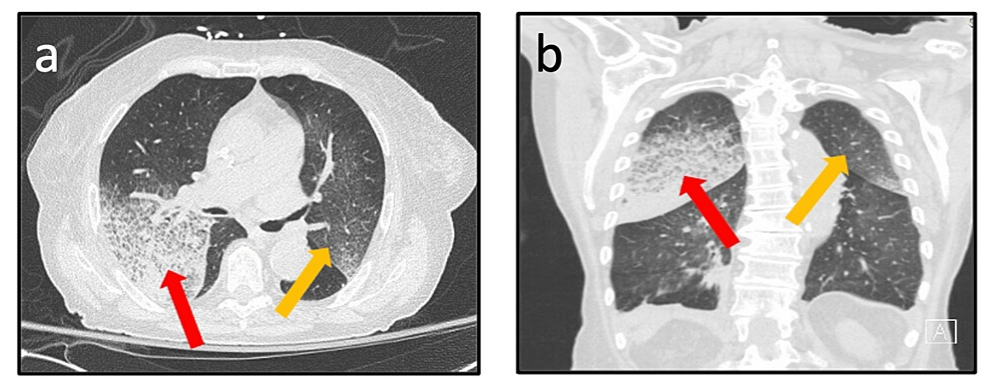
Coronal (a) and axial (b) chest CT demonstrating ground-glass attenuation superimposed on interlobular and intralobular lines in the right (red arrow) and left (orange arrow) upper lobes, an imaging pattern associated with parenchymal hemorrhage. CT: computed tomography
